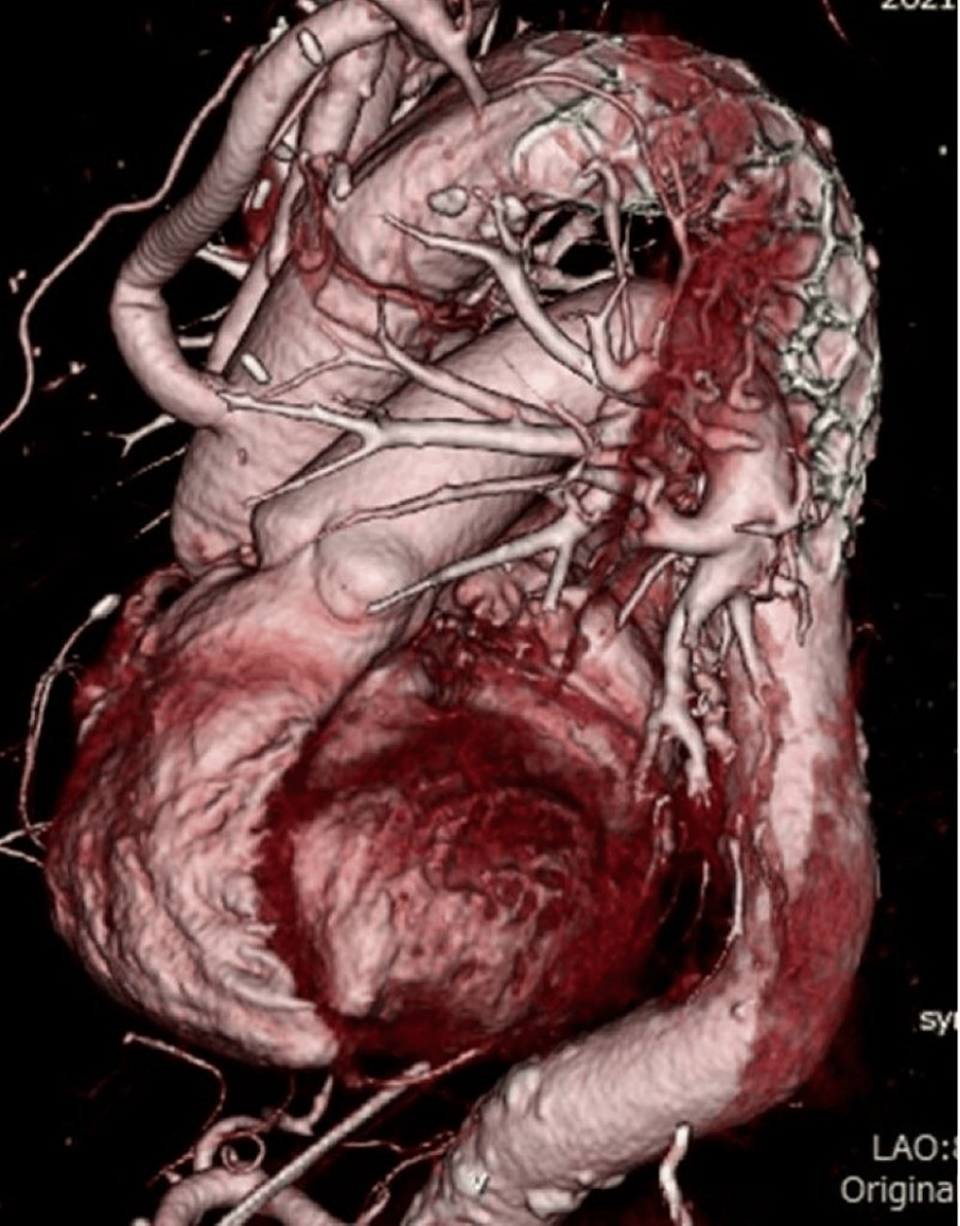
Postoperative computed tomography images No anastomotic abnormality was observed.
